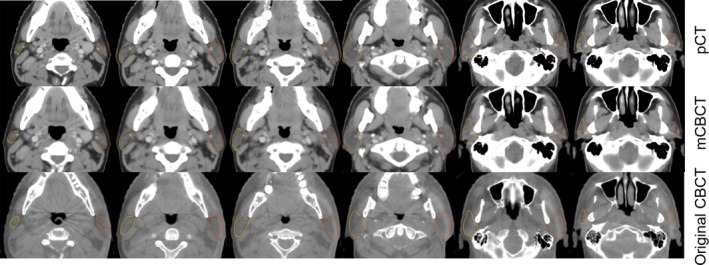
One example of automatically propagated parotid gland contours from planning CT to mCBCT. The last row shows the deformed contours overlaid on the original CBCT images. The yellow and brown line on CT and CBCT images represents the contour of the right and the left parotid gland respectively.
